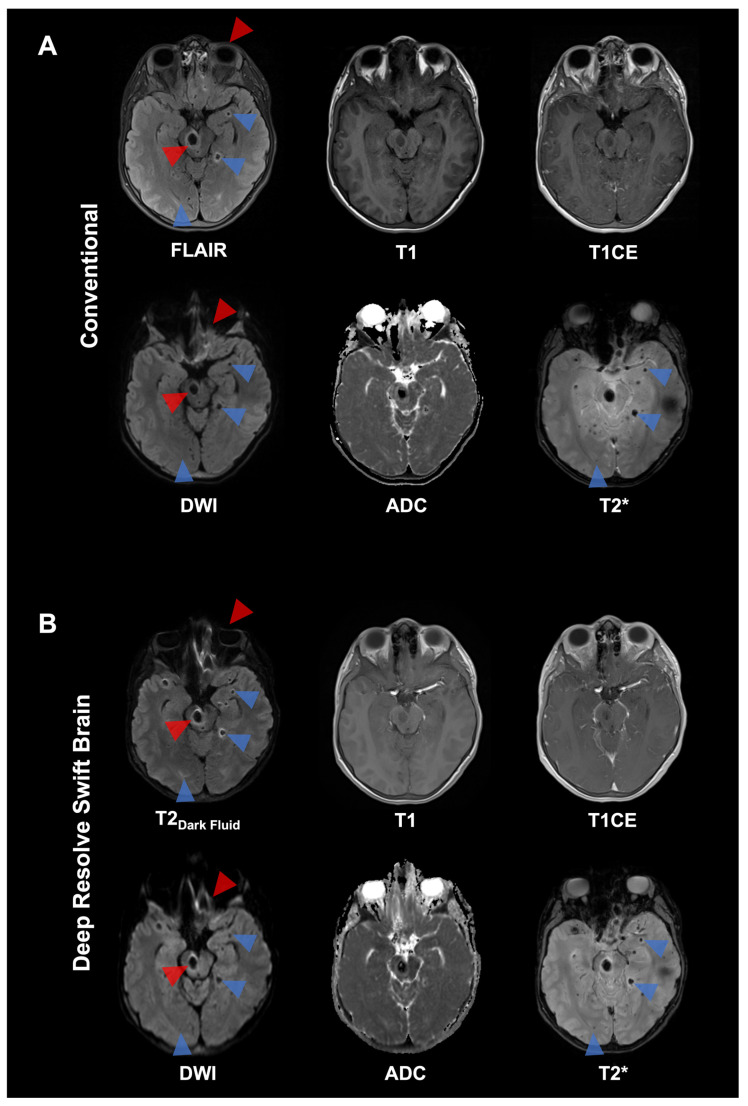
This 13-year-old male patient with a history of Ewing’s sarcoma presents with a leukostasis syndrome as a rare emergency related to hyperleukocytosis leading to microvascular occlusion and central nervous system (CNS) ischemia and bleeding (blue arrowheads). The top rows (A) show conventional MRI sequences, whereas the bottom rows (B) show the corresponding accelerated sequences using Deep Resolve Swift Brain. Due to alleviated EPI-related susceptibility artifacts and image distortions in T2-weighted dark fluid and DWI (red arrowheads), image quality is partially reduced in the Deep Resolve Swift Brain protocol in direct comparison. Brain MRI reveals small diffusion-restricted infarcts or microhemorrhages on T2*. CNS involvement can cause subtle signal changes that require careful review of DWI and T2* sequences (blue arrowheads) to detect early microinfarcts and -bleeds, which might be less visible on standard FLAIR and accelerated T2-weighted dark fluid sequences of the Deep Resolve Swift Brain protocol.
